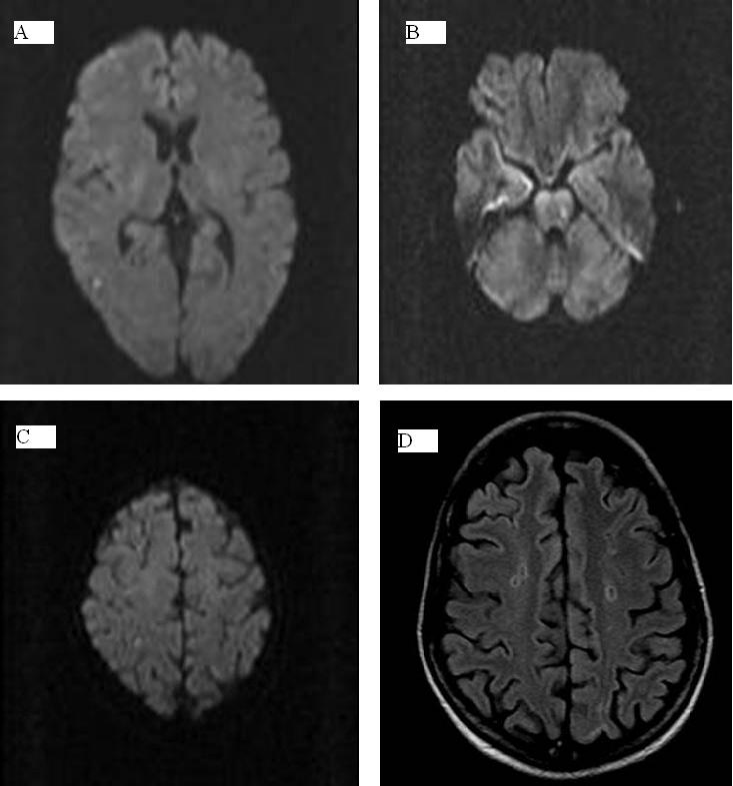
Other lesions suggestive of areas of acute embolic strokes are (A) the right parietal lobe, (B) the left pontine region and (C) the right parietal lobe (alternate view) . (D) Another bilateral centrum semiovale lesion not detected on diffusion-weighted images, without contrast enhancement, is hypointense in this fluid attenuated inversion recovery (FLAIR) image with a hyperintense gliotic peripheral ring, suggestive of small vascular ischemic microlacunae.
